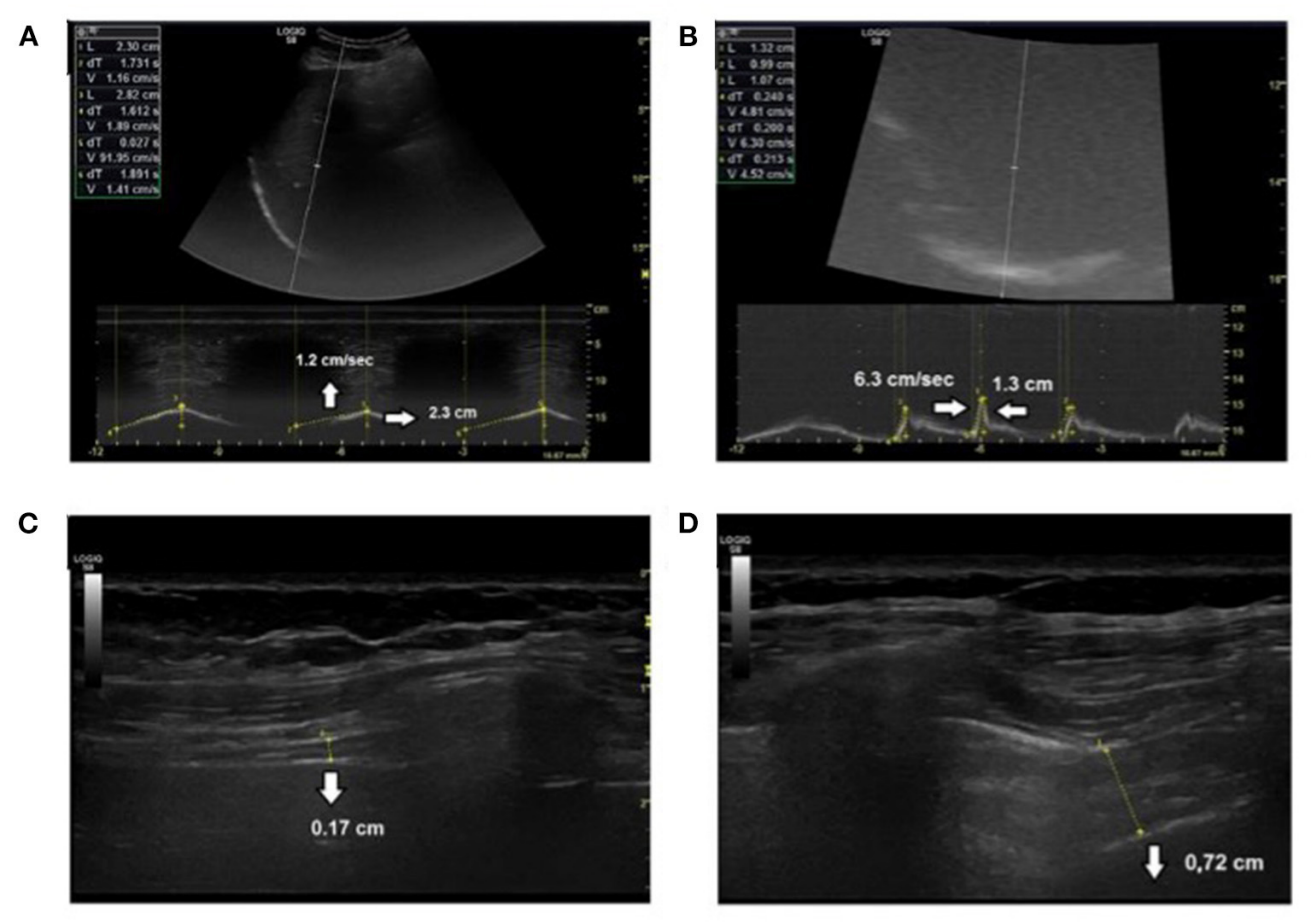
Diaphragm ultrasound measures. (A) Diaphragm excursion amplitude and velocity during tidal breathing, (B) diaphragm excursion amplitude and velocity during voluntary sniff, (C) diaphragm thickness at functional residual capacity, and (D) at total lung capacity.
